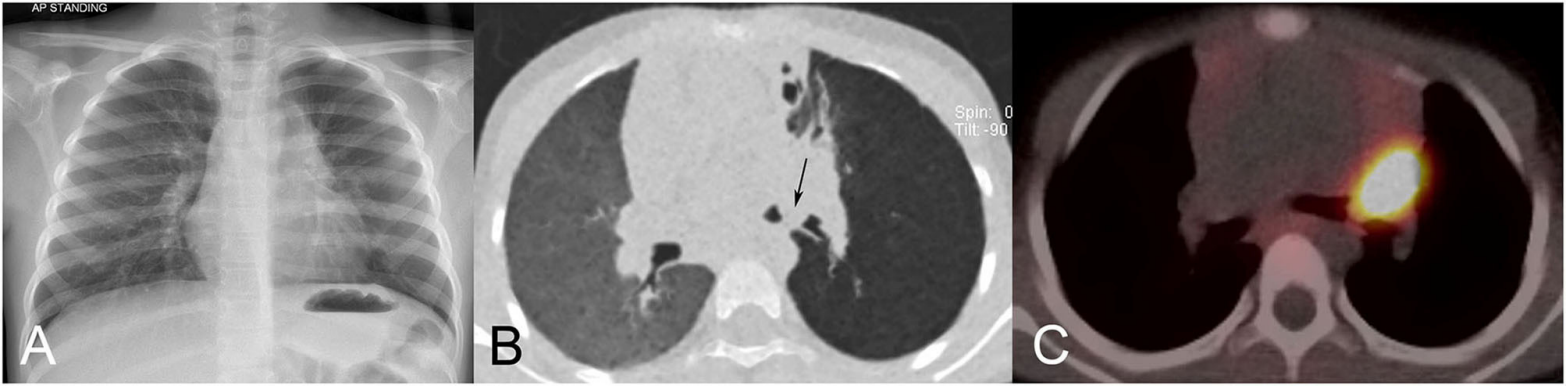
Carcinoid tumor in a 6-year-old-girl. (A) Chest radiograph shows a left hilar mass and hyperlucency of the left upper lobe. (B) Minimum intensity projection (MinIP) CT image shows a soft tissue mass occluding the left upper lobe bronchus, and protruding into the left bronchial bifurcation (arrow). Note the striking hypoattenuation (hyperlucency) of the left lung. (C)
